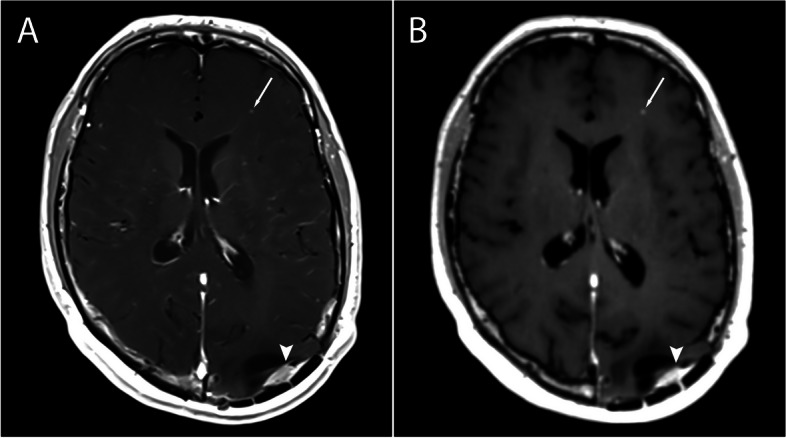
A T1-weighted SE sequence. B T1-weighted SPACE sequence. Small metastasis in the left frontal lobe (arrow) in a 53-year-old female at 3 T, Gadovist 0.1 mL/kg. Postresection changes with an implantation metastasis in the left occipital region (arrowhead). The SE sequence was acquired first
